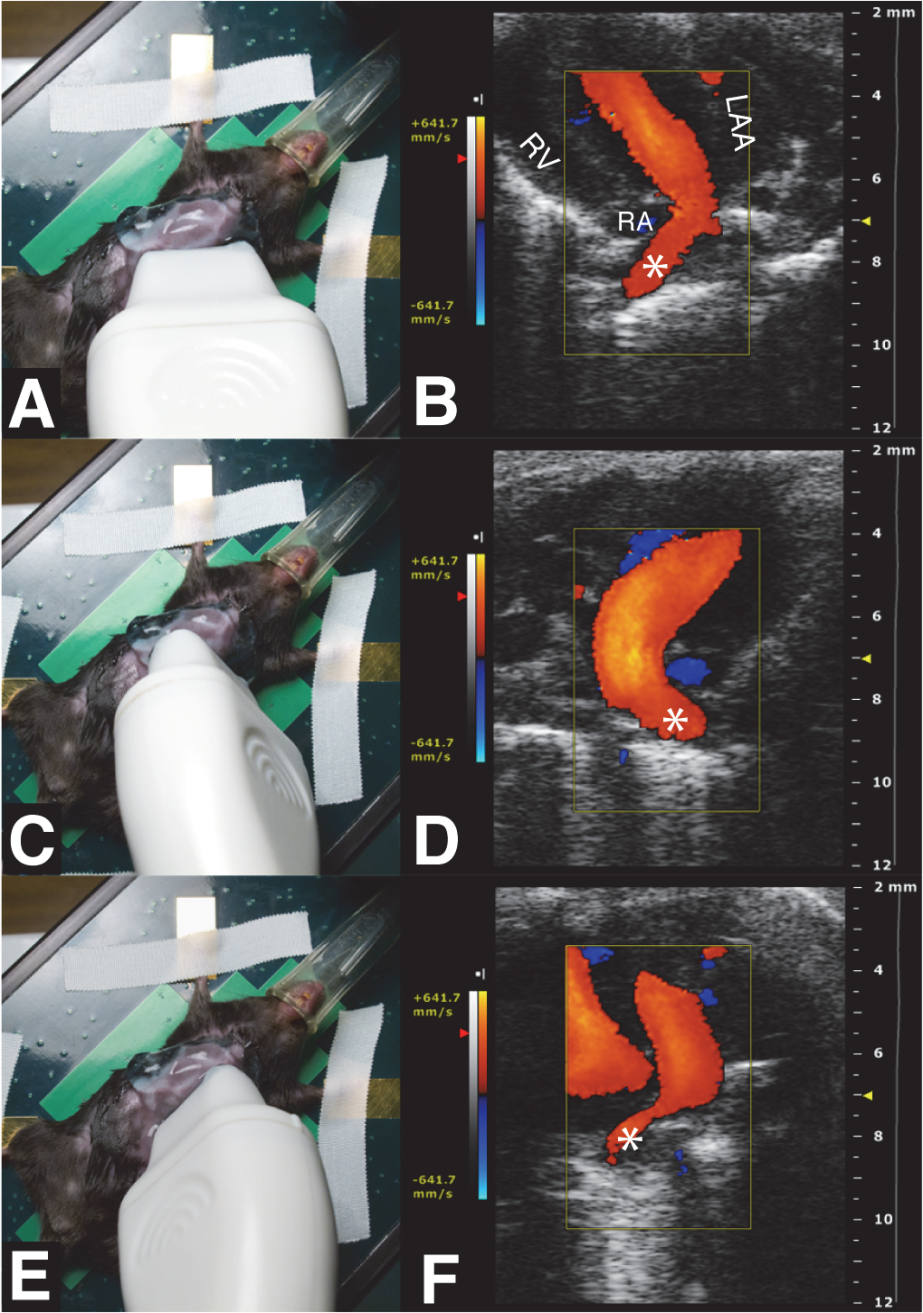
Color Doppler and anatomy of the three pulmonary veins. A) Modified apical 4-chamber view to visualise the right superior PV. B) Color Doppler LA inflow from the right superior PV. C) Transducer rotated clockwise to visualise left PV. D) Color Doppler LA inflow from the left PV. E) Transducer rotated counter-clockwise to visualise right inferior PV. F) Color Doppler LA inflow from right inferior PV. *: PVs; LAA: left atrial appendage; RA: right atrium; RV: right ventricle.
